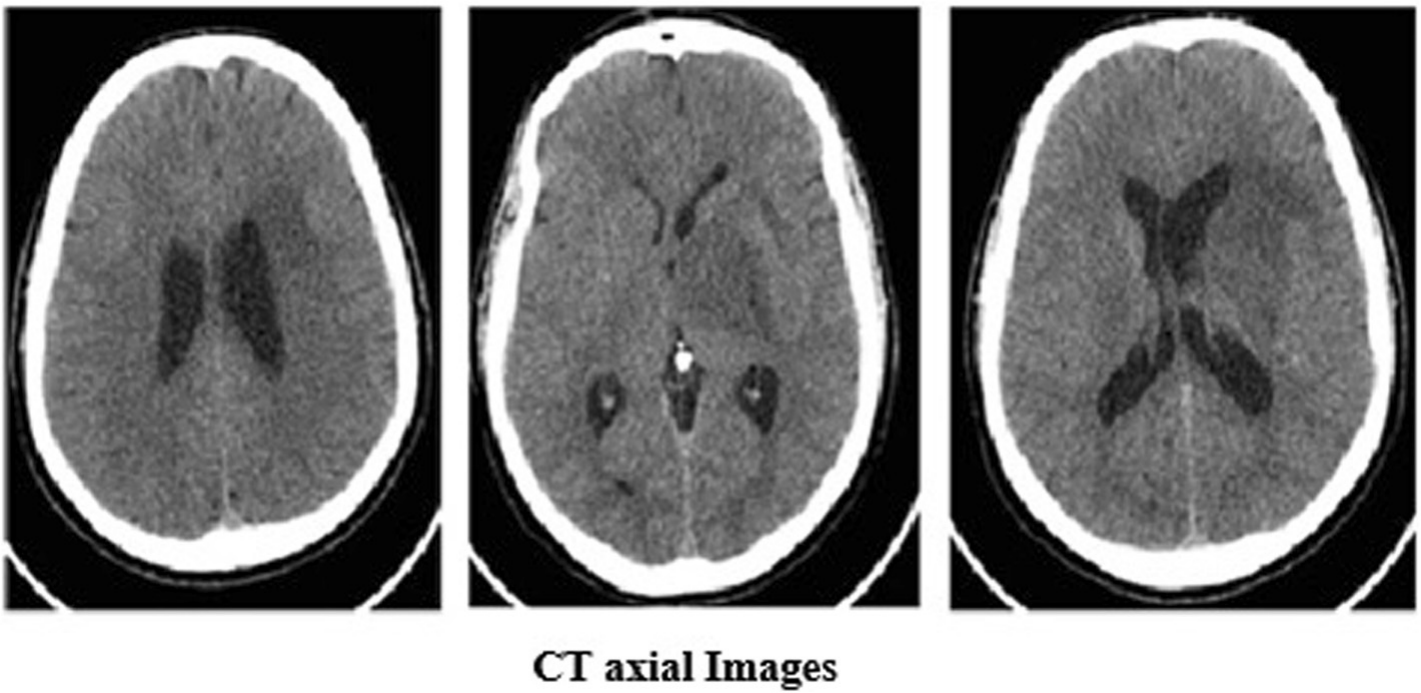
Brain computed tomography (axial images). Left temporoparietal hypodensity extending into left cerebral peduncle with faint central hyperdensity with mass effect and midline shift of 5mm
